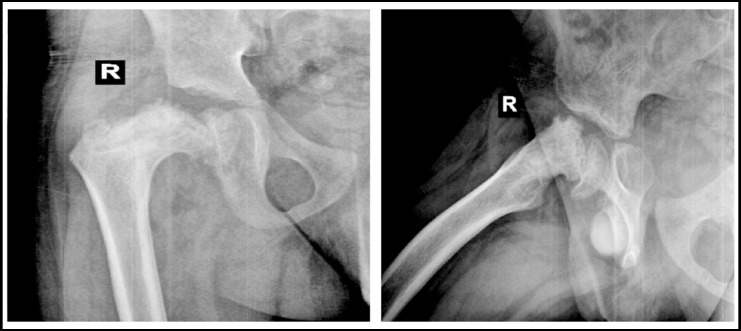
X-rays after 7 months of spica.
